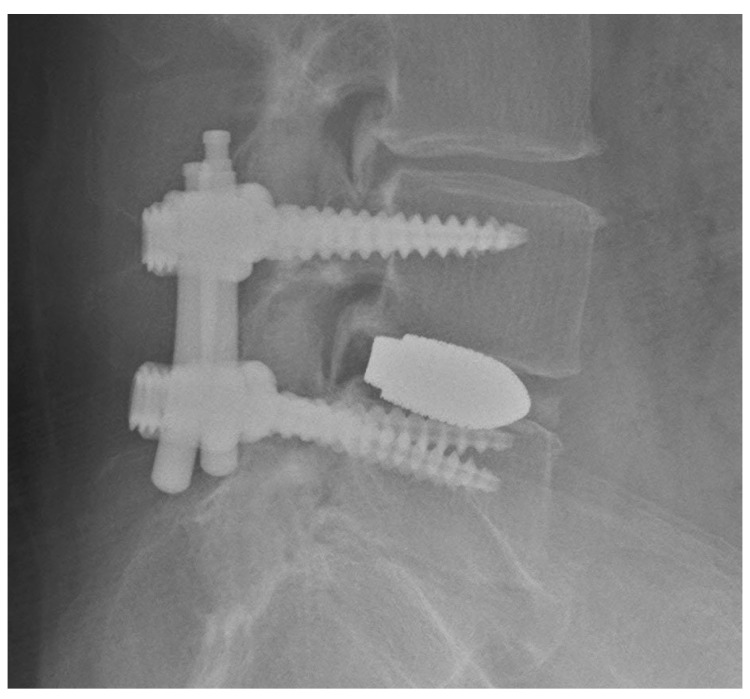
Lateral radiograph of ossification of the posterior longitudinal ligament of the lumbar spine.
